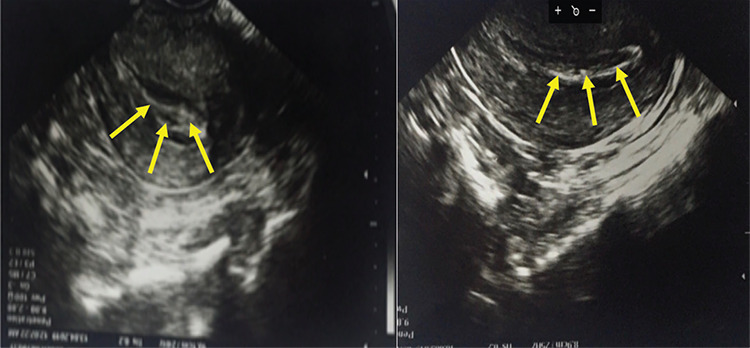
Colposcopic and ultrasound examination of the uterus revealed a woody membranous lesion that covered the inner part of the uterine cavity; a regular cavity view was achieved after treatment.
